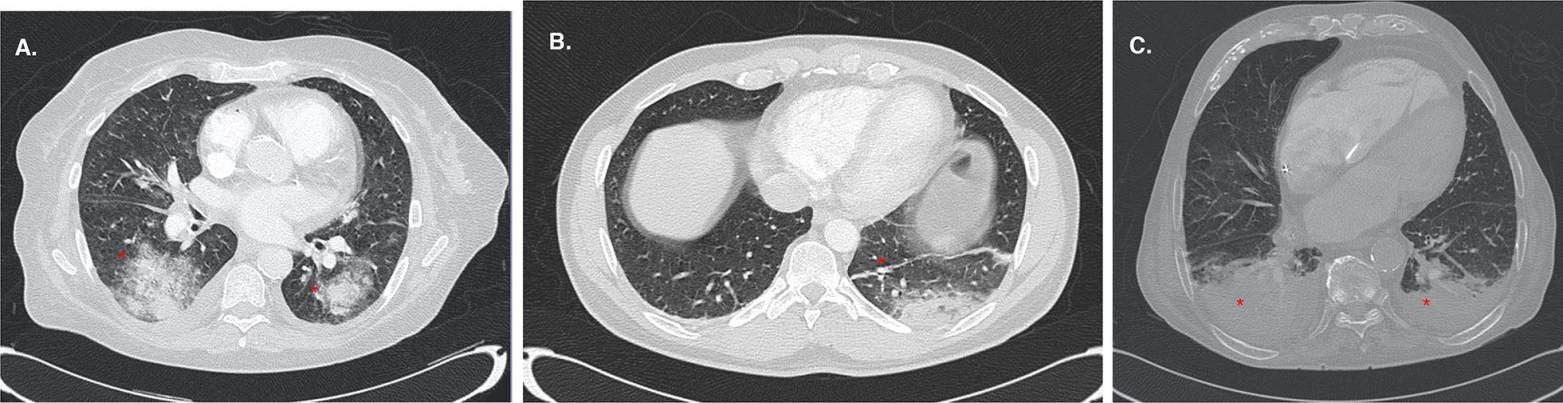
Representative chest Computed Tomography scans in patients with SARS-CoV-2 pneumonia. a Patient without atelectasis: multiple ground-glass opacities in both lungs (red asterisks), as well as a minimum pleural effusion that do not condition atelectasis. b Patient with small atelectasis. Well-defined, linear opacity is seen in the left lower lobe (red asterisk), corresponding to laminar atelectasis (middle panel). c Patient with large atelectasis. A triangular opacity in both inferior lobes (red asterisk) corresponding to atelectasis is observed, surrounded by pleural effusion in the same location (right panel)
